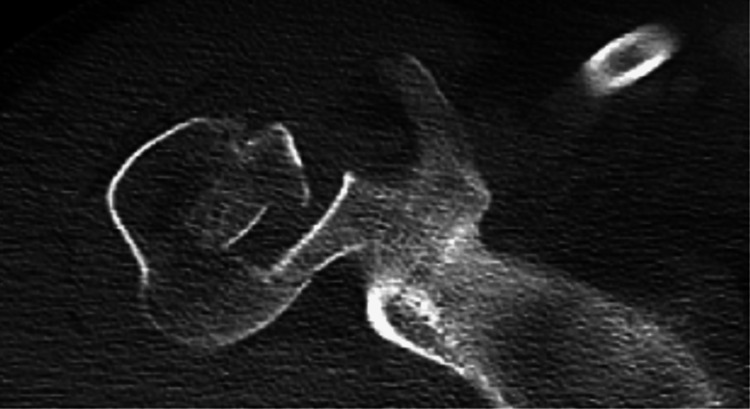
CT scan depicts the extent of the defect.
